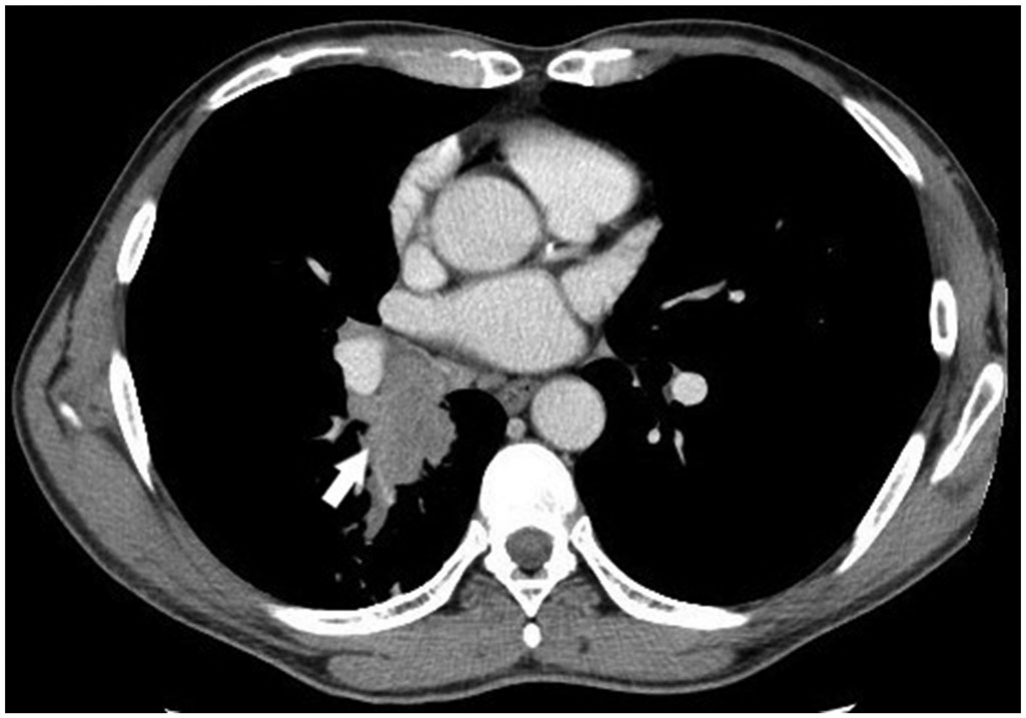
Thoracic CT scan image at the time of diagnosing SqCLC. Contrast-enhanced CT image with soft-tissue window showing a centrally located mass in the right lung with mediastinal invasion (arrow), and mediastinal lymphadenopathy.
